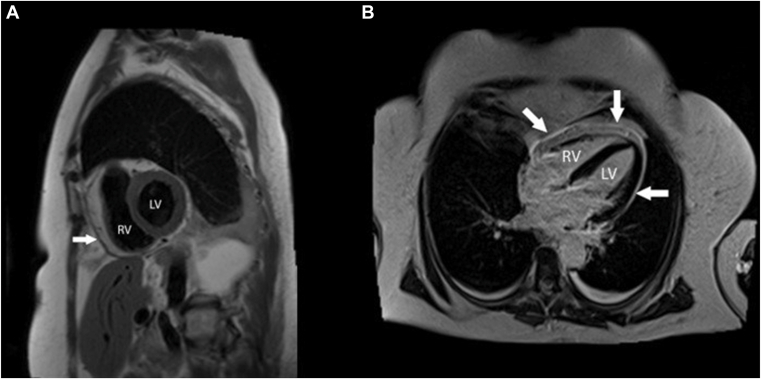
Cardiac magnetic resonance findings. (A) Short-axis T1-weighted turbo spin-echo image shows a thickened (4 mm) pericardium overlying the right ventricle (RV; arrow). (B) Four-chamber T1-weighted gradient echo late gadolinium enhancement image demonstrates circumferential enhancement of the pericardium (arrows). LV, left ventricle.
